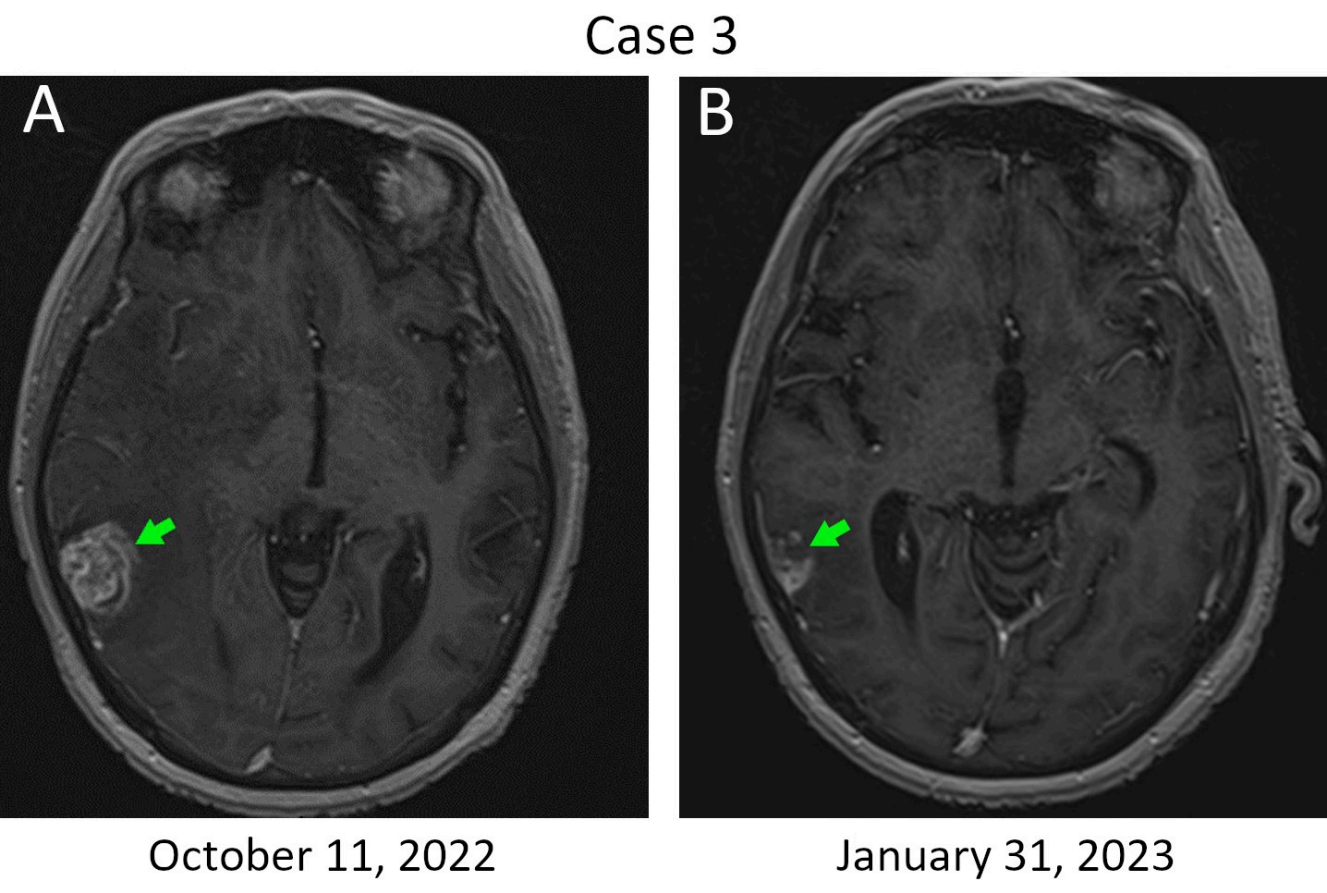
The response of metastatic lesions located in the right temporal lobe to a single-agent bevacizumab therapy. (A) Metastatic lump at baseline. Bevacizumab therapy was started on December 8, 2022; (B) MRI imaging was performed on day 54 after the initiation of this treatment. The baseline size of the metastasis is 24 mm × 21 mm (green arrow, left), while a 45% reduction in the tumor size was observed in the next examination (13 mm × 12 mm; green arrow, right)
